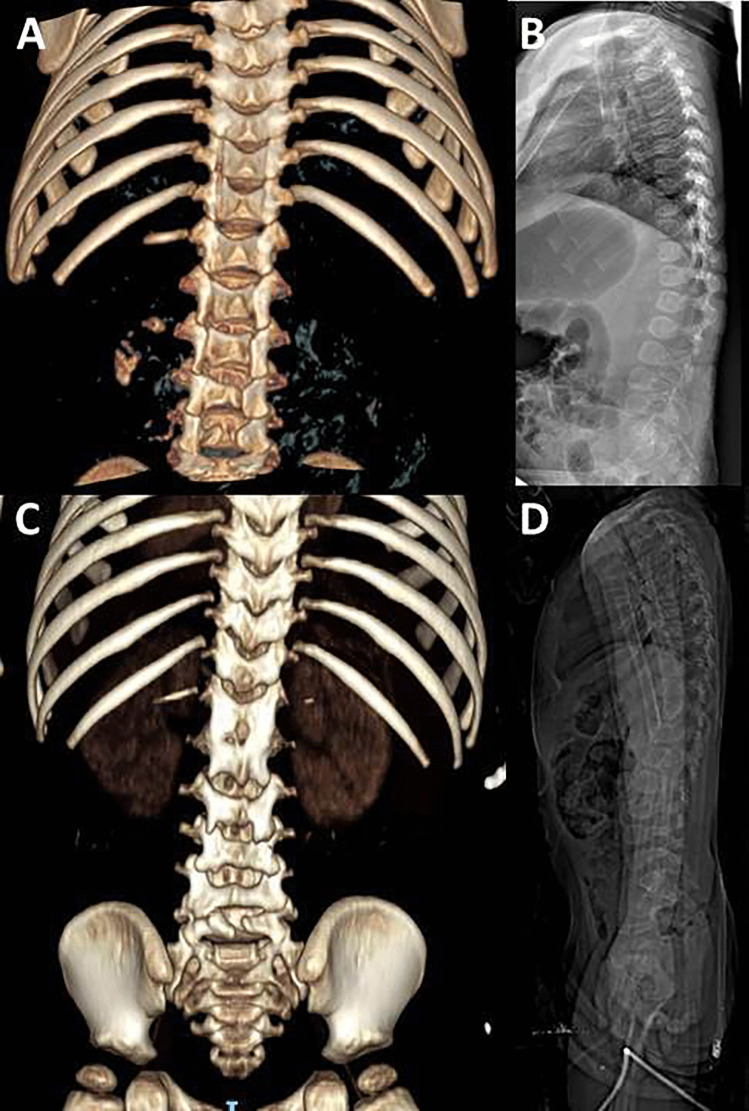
3D-CT scan and lateral X-rays of the spine confirming the optimal reconstruction of the laminae in the immediate postoperative follow-up (A and B, respectively) and fusion without spinal deformity after 1 year (C and D, respectively)
